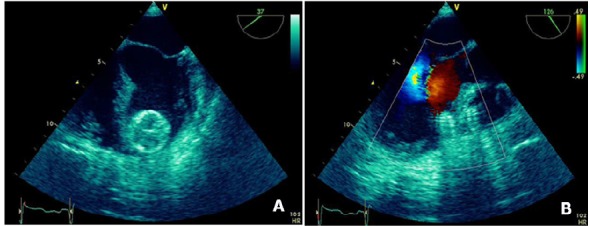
Both TEE (Figure 2B) and left ventriculography (Figure 3) confirmed good placement of the device with no detectable residual shunt, demonstrating good device size selection. The patient showed immediate significant symptomatic improvement. He was transferred to the coronary care unit, and discharged seven days later. As of the date of this case report, the patient is alive and feeling well with no residual shunt detected by transthoracic echocardiography. No procedure-related complications have been recorded during these six years.
